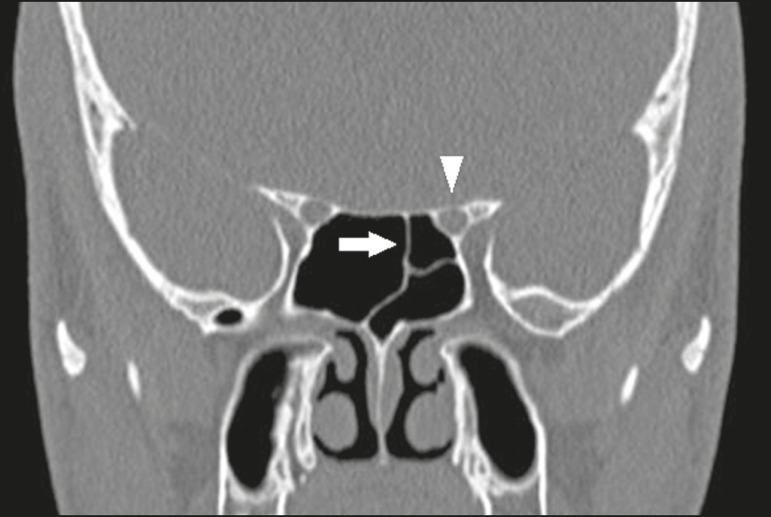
Coronal CT slice, with bone window settings, showing the left
